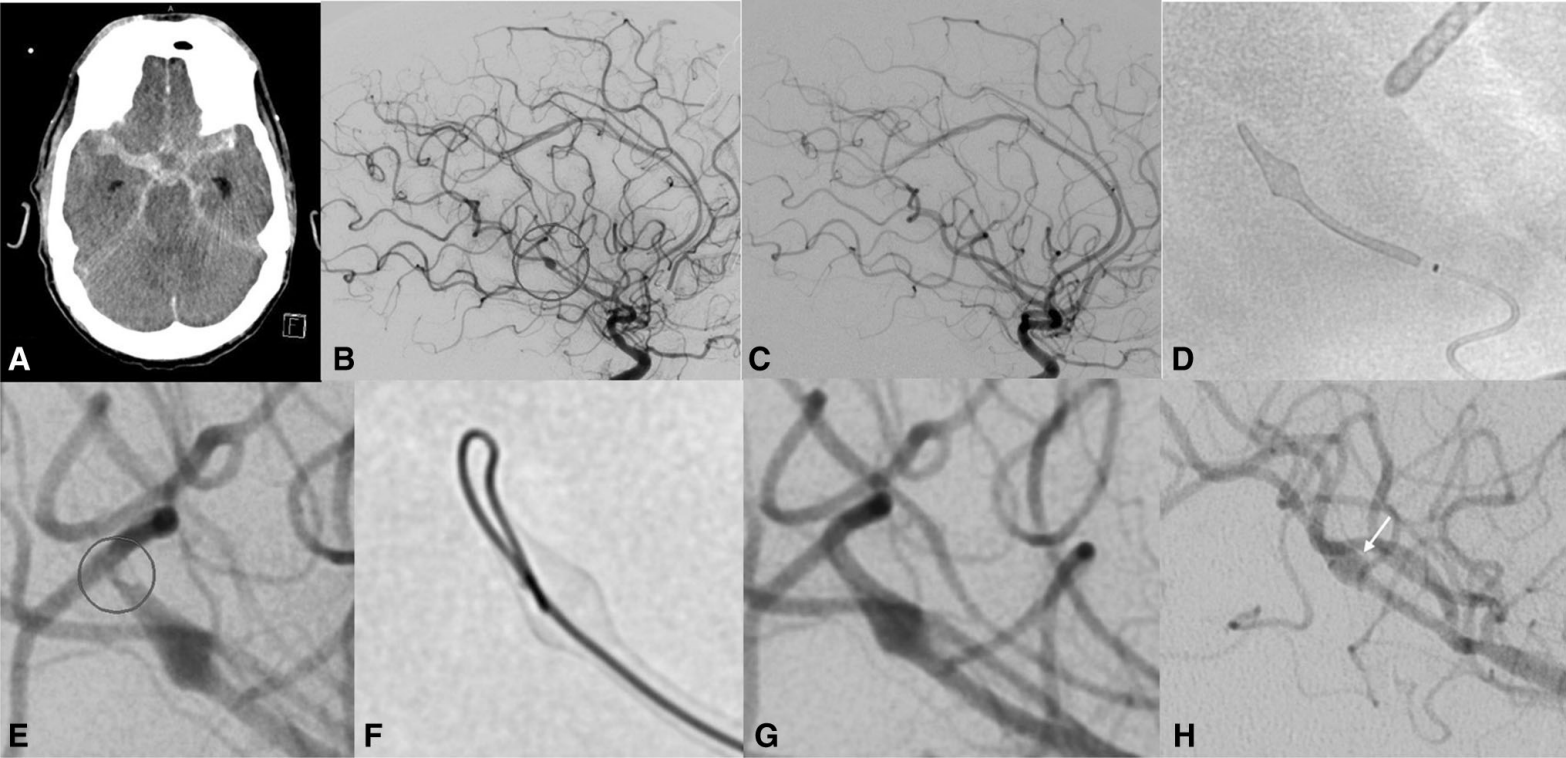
A 50-year-old and drug-addicted male presented with subarachnoid hemorrhage from a left mycotic MCA aneurysm, who was treated by parent vessel occlusion (Patient #6). Four days later, and with the patient still intubated, he presented a sudden increase in the intracranial pressure. Head CT scan revealed a second subarachnoid hemorrhage, more prominent on the right side (A). A new diagnostic angiography showed a de novo right M2 mycotic aneurysm (B, circle), which was not present in the initial angiography (C). A single p48MW HPC (3 mm × 18 mm) was implanted after premedication with ASA (D). A total of 1500 mg ASA IV was needed to achieve sufficient platelet inhibition, tested by Multiplate and VerifyNow. After deployment, an incomplete opening of the distal end of the p48MW HPC with small thrombus formation was observed (E, circle). The complete opening of the device was achieved after crossing with an inverted microguidewire (F). The thrombus formation resolved after injection of eptifibatide (G). The most recent follow-up angiography performed 8 months after treatment showed the p48MW HPC following the contour of the fusiform vessel enlargement (H, arrow)
